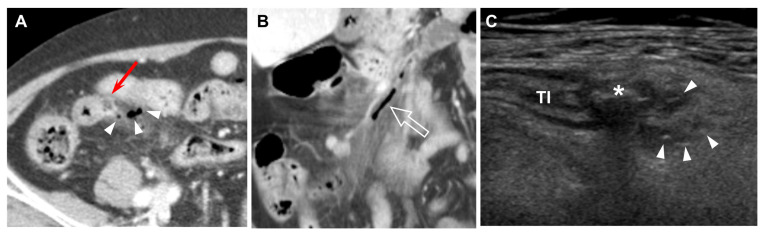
Sixty-three-year-old female (patient #9) with acute perforated ileal diverticulitis. (A,B) Axial and coronal reformatted CT scans show inflamed diverticulum (red arrow), with extraluminal gas (arrowheads) representing perforation. Mesenteric fat infiltration, terminal ileal wall thickening, and mesenteric venous gas (open arrow) are also seen. (C) US scan demonstrates an outpouching inflamed diverticulum with central hyperechoic fecalith (*) connecting to terminal ileum (TI), and tiny hyperechoic reverberating dots (arrowheads) represent extraluminal air bubbles.
